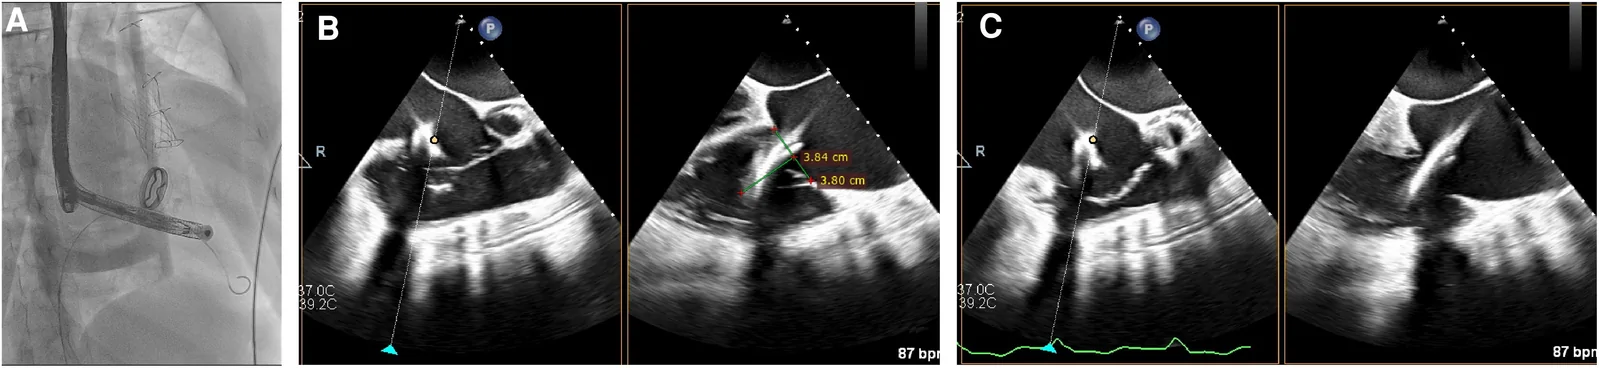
Intraprocedural multimodality imaging during transjugular LuX-Valve Plus deployment. (*A*) Fluoroscopy showed the delivery-system trajectory; the radiopaque mechanical mitral prosthetic frame served as a landmark for annular localization and system orientation. (*B*, *C*) Transoesophageal echocardiography showed the delivery system–annulus relationship for depth assessment and confirmation of coaxial alignment.
